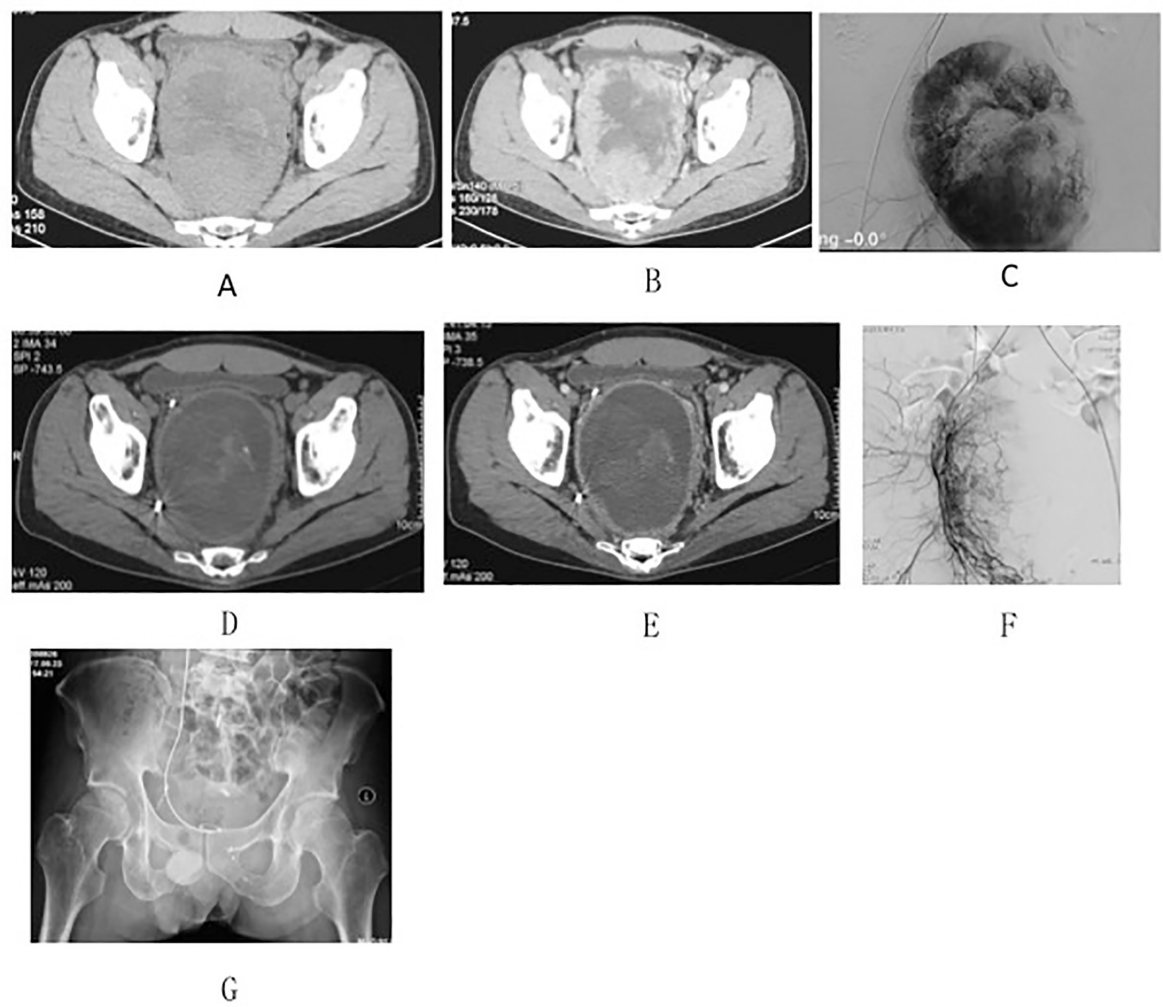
(A-G) A 38-year-old male patient with sacral primary solitary fibrous tumor received oral administration of apatinib 750 mg qd treatment together with two courses of selective arterial embolization at our hospital, followed by surgical treatment. Preoperative plain scan (A), enhanced CT (B), and angiography (C). The tumor was mainly solid component-based. Enhanced CT showed that the solid component was significantly enhanced, and angiography suggested that the tumor received an abundant blood supply. Plain scan (D), enhanced CT (E), and angiography (F) after treatment. The tumor size did not change significantly compared with that before treatment. However, the inside of the tumor mainly consisted of liquefied and necrotic regions, with solid components located only in the peripheral areas of the tumor. Enhanced CT showed only enhancement of the peripheral region. Angiography showed that the blood supply of the tumor had been reduced significantly. Postoperative pelvic anteroposterior X-ray film showing the low sacrococcygeal bone resection (G).
